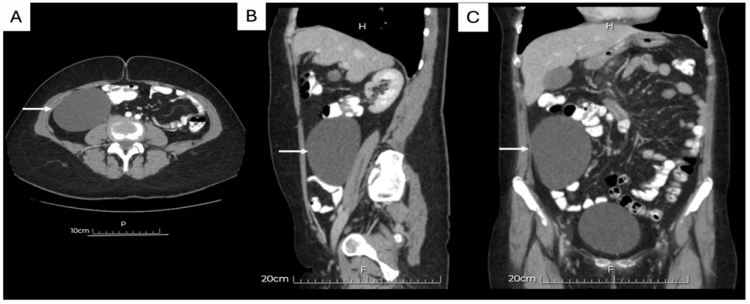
CT imaging of the retroperitoneal mass. Abdominal computed tomography in the (A) axial, (B) sagittal, and (C) coronal planes shows a large retroperitoneal cystic mass (arrows) with regular borders and no intracystic structures.
